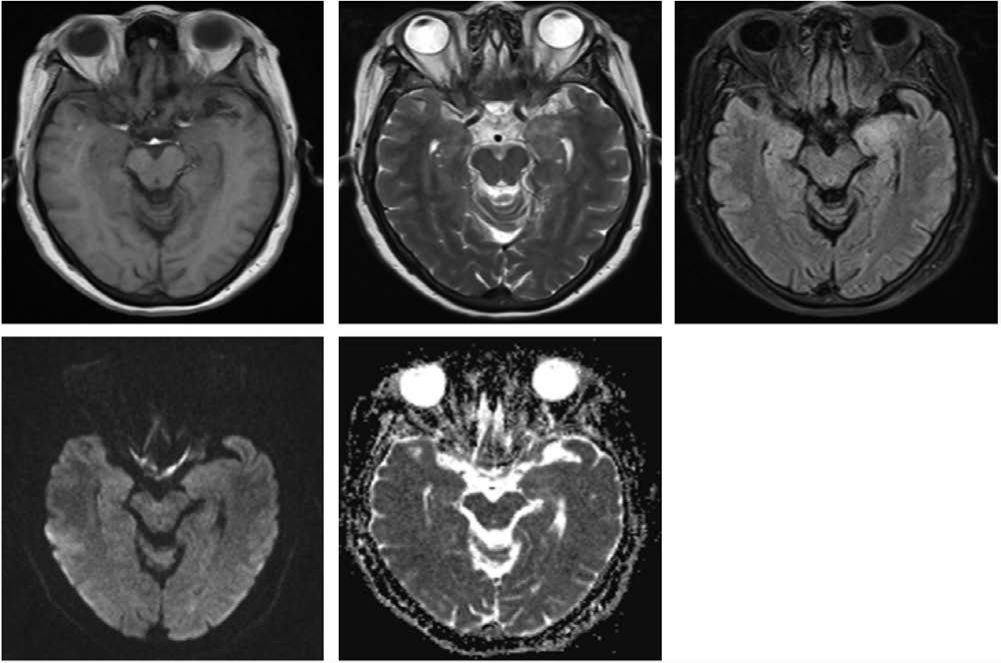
The left hippocampus showed slightly longer T1 and T2 signal plaques, slightly higher signal on FLAIR sequence, no diffusion limitation on DWI, slightly higher signal on ADC. ADC = apparent diffusion coefficient, DWI = diffusion-weighted imaging, FLAIR = fluid attenuated inversion recovery, T1 = T1-weighted imaging, T2 = T2-weighted imaging.
